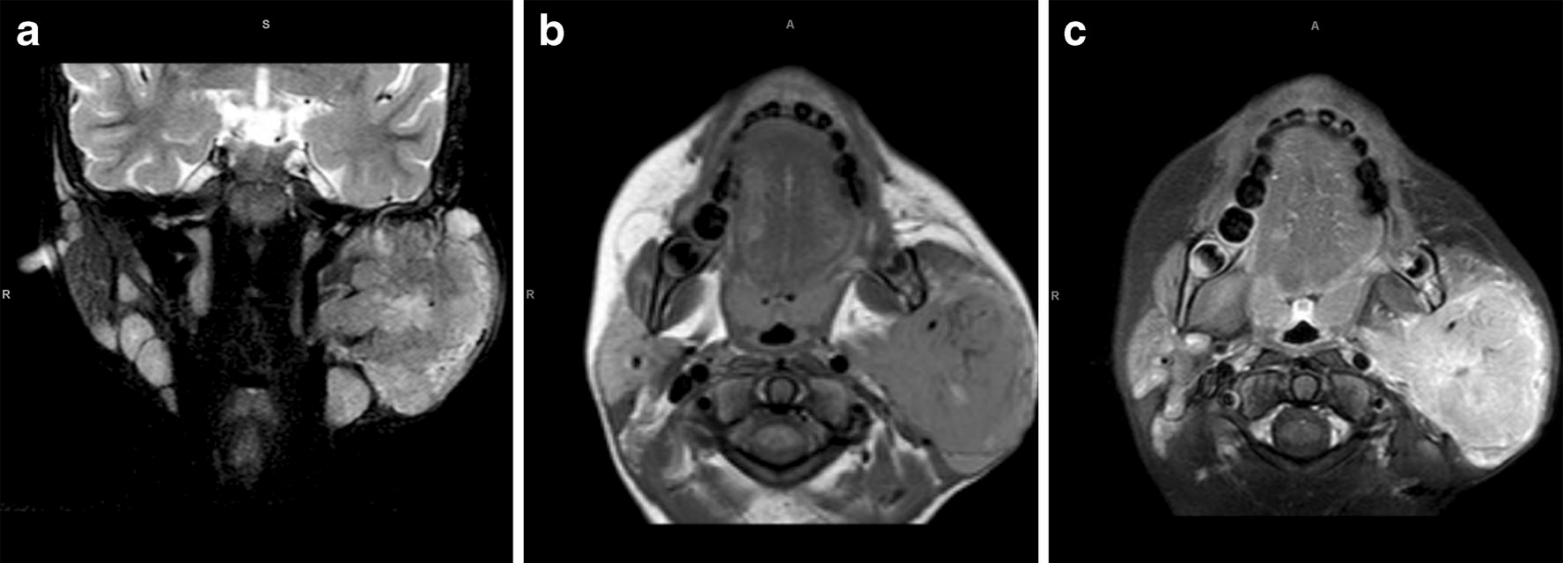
Mucoepidermoid carcinoma. Coronal T2-W MRI (a) shows a hyperintense, well-defined left intra-parotid mass which is iso- to hyperintense on the axial T1 W view (b), and enhances avidly post-contrast (c). There are intratumoral cysts and necrosis, as well as associated bilateral cervical and posterior triangle lymphadenopathy
